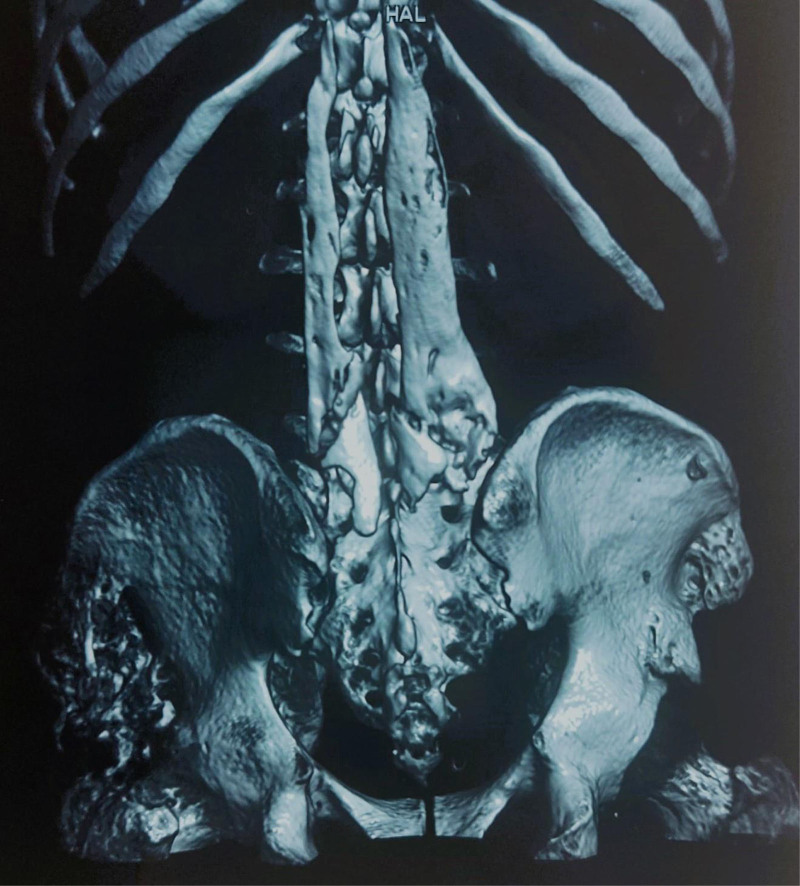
CT 3-dimensional reconstruction of spinal muscles and hips. CT = computed tomography.
